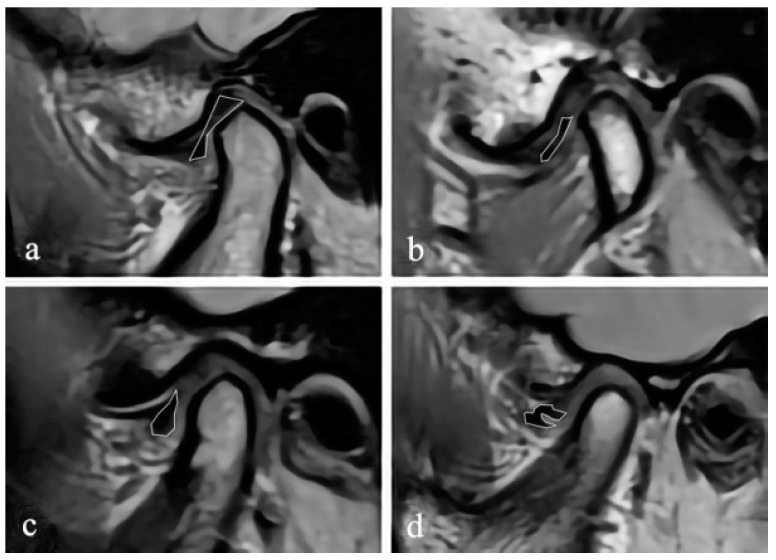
Disc configuration. Oblique sagittal proton density MRI showing the classification of disc configuration: (a) biconcave, (b) biplanar, (c) convex, and (d) folded.
